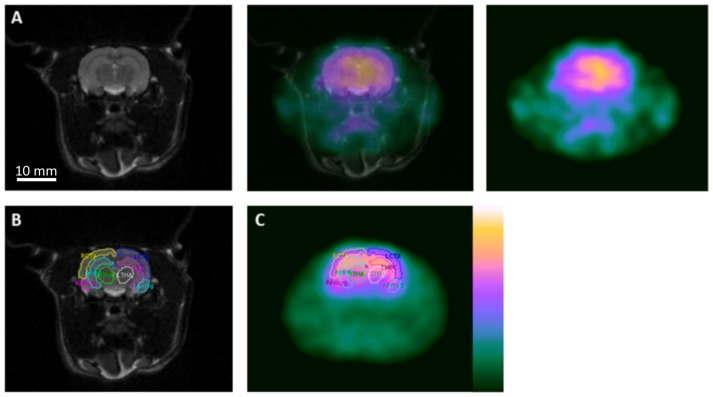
Coregistration and region of interest delineations for image analysis. (A) Coregistration of [^18^F]-FMZ PET with T2 weighted MRI, (B) Delineation of regions of interest on MRI (RCTX right cortex, LCTX left cortex, RHPC right hippocampus, LHPC left hippocampus, RTHA right thalamus, LTHA left thalamus, RAmyg right amygdala, LAmyg left amygdala), (C) Application of regions of interest on to [^18^F]-FMZ PET image.
